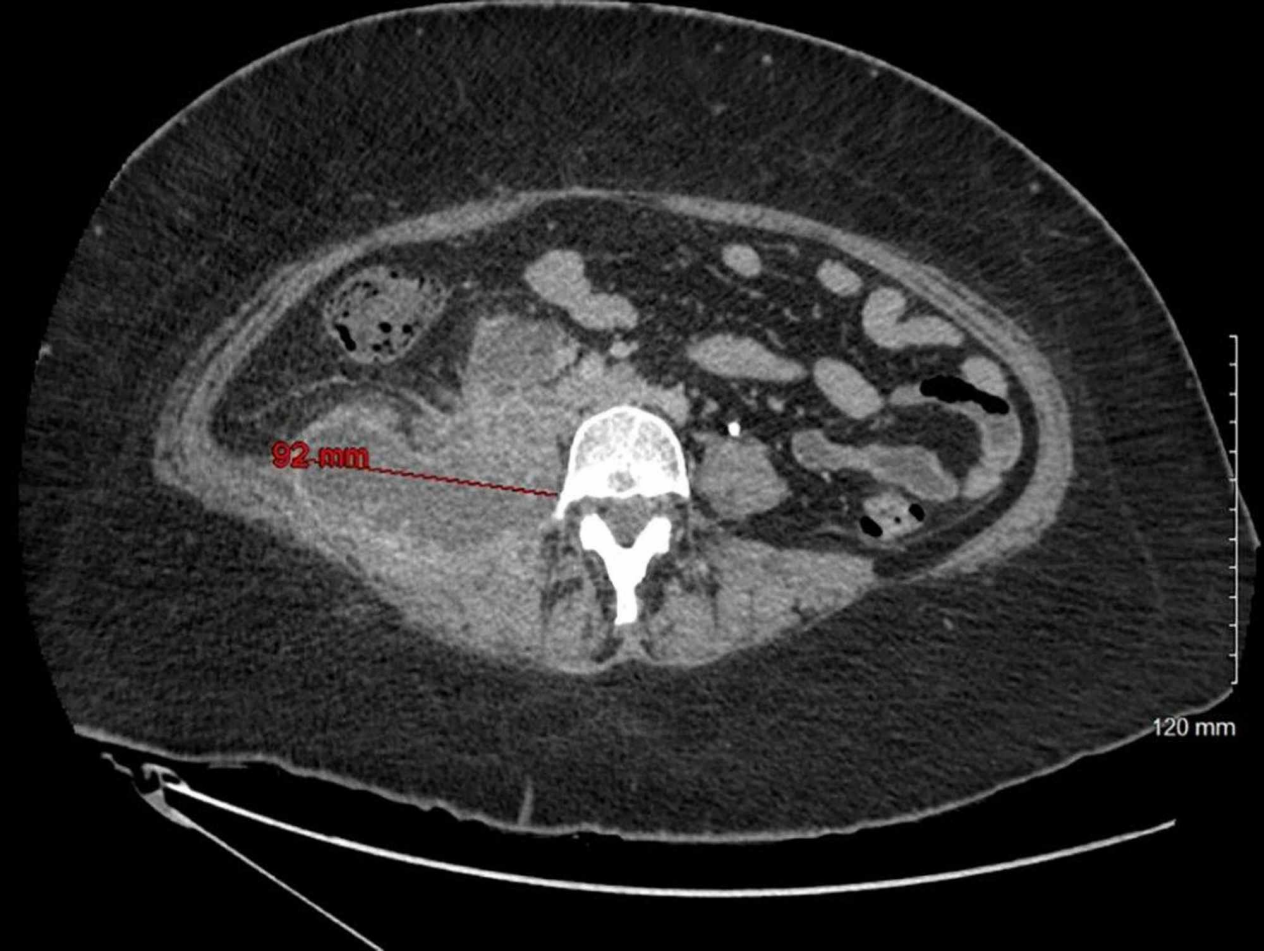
CT abdomen/pelvis with contrast on admission Multiloculated abscess 8 x 9 x 9 cm in the right psoas and quadratus lumborum musculature CT: computed tomography
